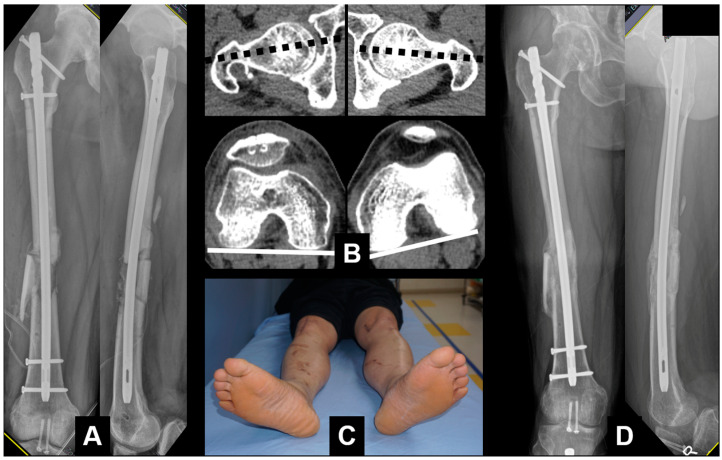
(A) Postoperative radiographs showing antegrade nailing with bone graft at the nonunion site; (B) CT scan showing similar angles of anteversion; (C) similar rotation in foot position; (D) complete healing observed 1 year postoperatively.
